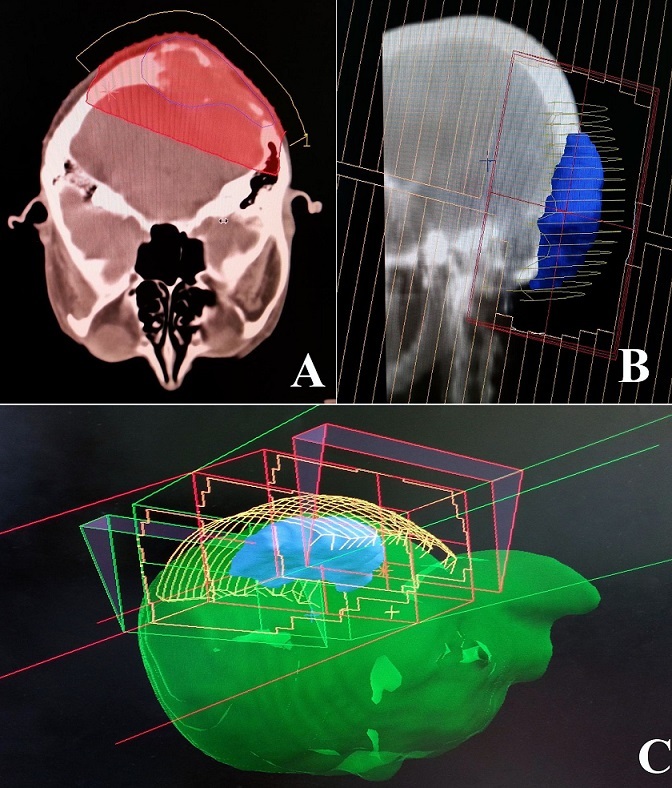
Images issues du logiciel de planification de traitement pour radiothérapie. A. Coupe scannographique axiale montrant le volume cible (Contour bleu) couvert par l'isodose 95% (Aire rouge). B. Radiographie reconstruite montrant un des deux champs de traitement. C. Modèle 3D généré montrant la balistique du traitement
